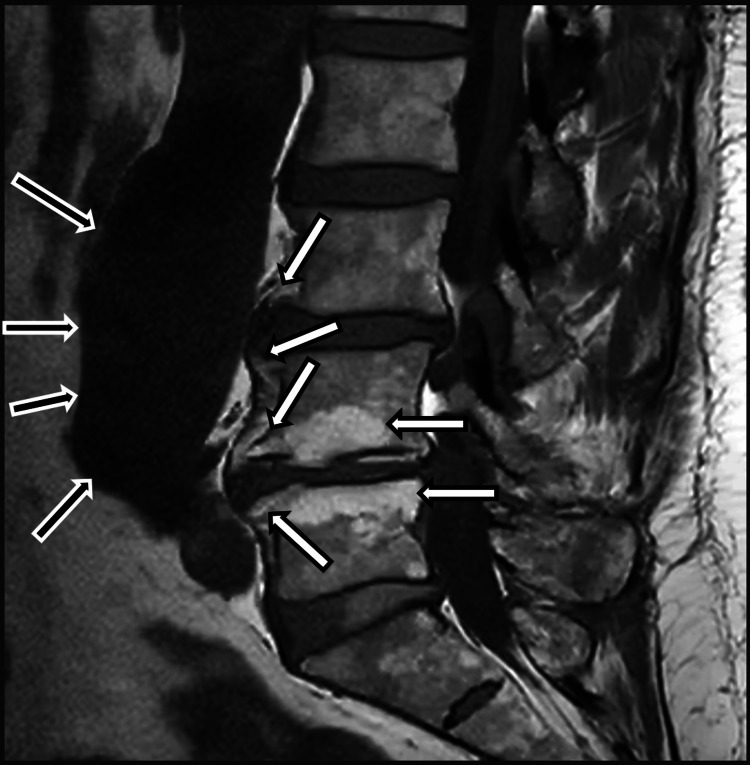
MRI T1 acquisition of the lumbar spine from subject "iv-377". The white arrows point out multiple vertebral modifications such as osteophytes and fatty infiltration of the vertebral plateaus. The black arrows identify an aneurysmal dilation of the abdominal aorta, associating parietal thrombosis. MRI, magnetic resonance imaging.
